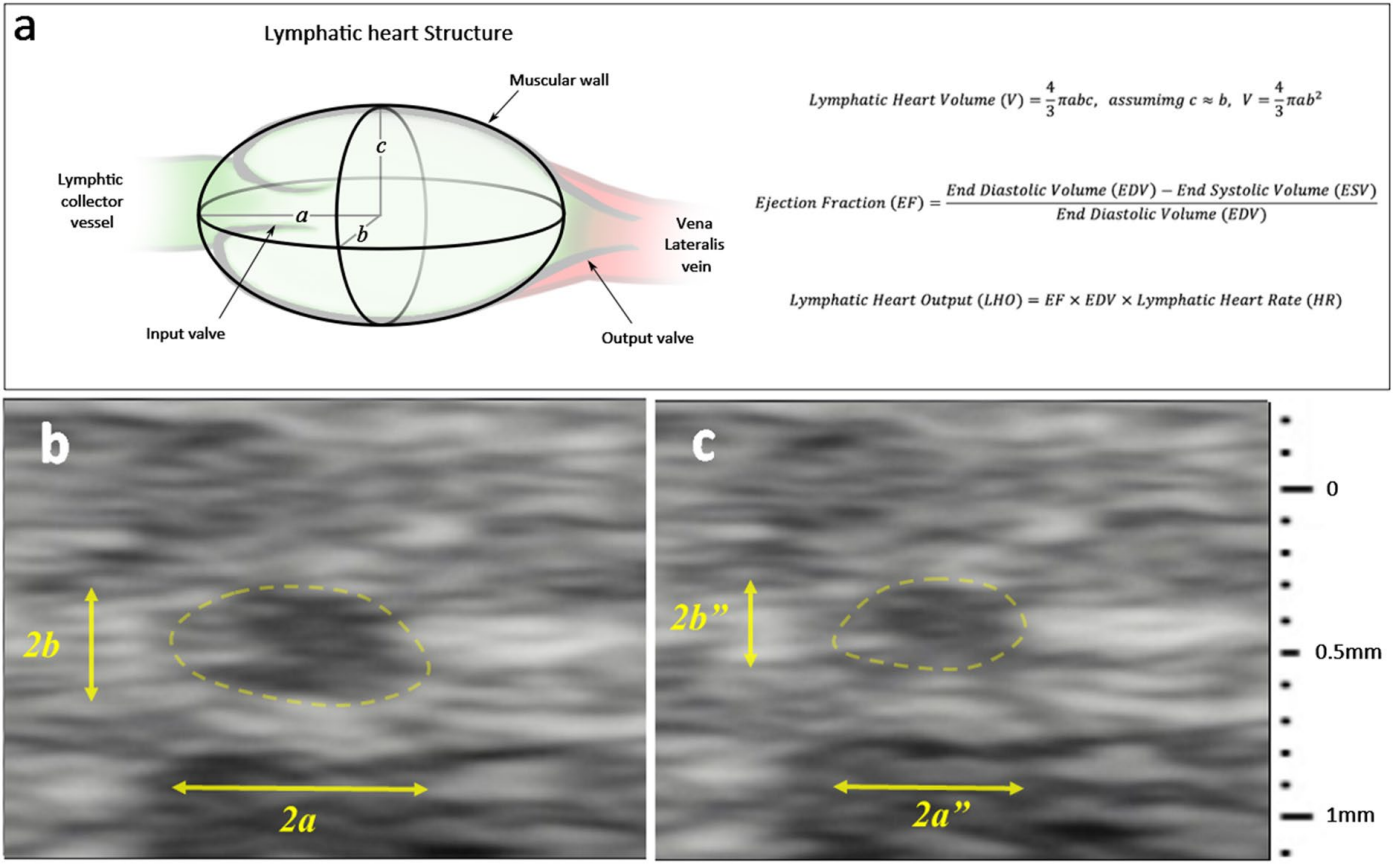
Ultra-high frequency ultrasound-based calculation of the newt lymphatic heart cardiac output. (a) The lymphatic heart (LH) structure consists of a single muscular chamber receiving lymphatic fluid from the lymphatic collectors and pumping this fluid into the vena lateralis vein. The LH has one input valve and one output valve that prevent backflow of blood. Computer 3D volume reconstruction showed the newt lymphatic heart shape conformed best to an ellipsoid-like shape with a slightly pointed output end and flattened input end, therefore, the ejection fraction (EF) was calculated using an ellipsoid mathematical model based on 2D ultra-high frequency ultrasound measurement of the LH end diastolic volume (EDV) (b) and end systolic volume (c), and the LH rate. The mean EF was 60.40% (SD 12.94), EDV 0.026 mm^3^ (SD 0.008) and the mean lymphatic heart rate was 117.04 bpm (SD 23.12), giving a LH cardiac output (LHO) of 1.838 mm^3^/min for each LH and a combined total of 58.816 mm^3^/min for all 16 pairs.
